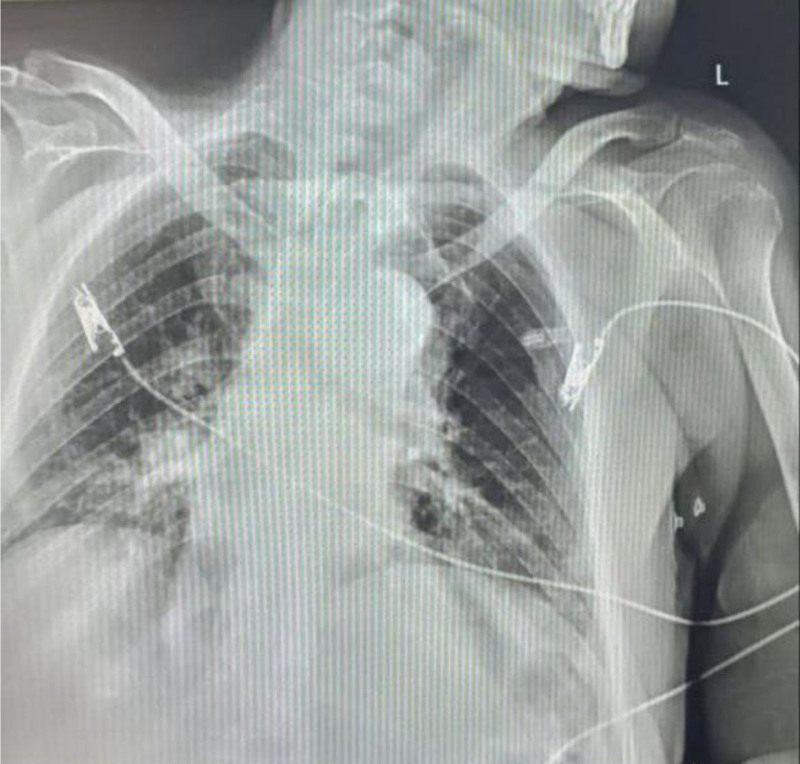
Post-procedural chest X-ray scan.
